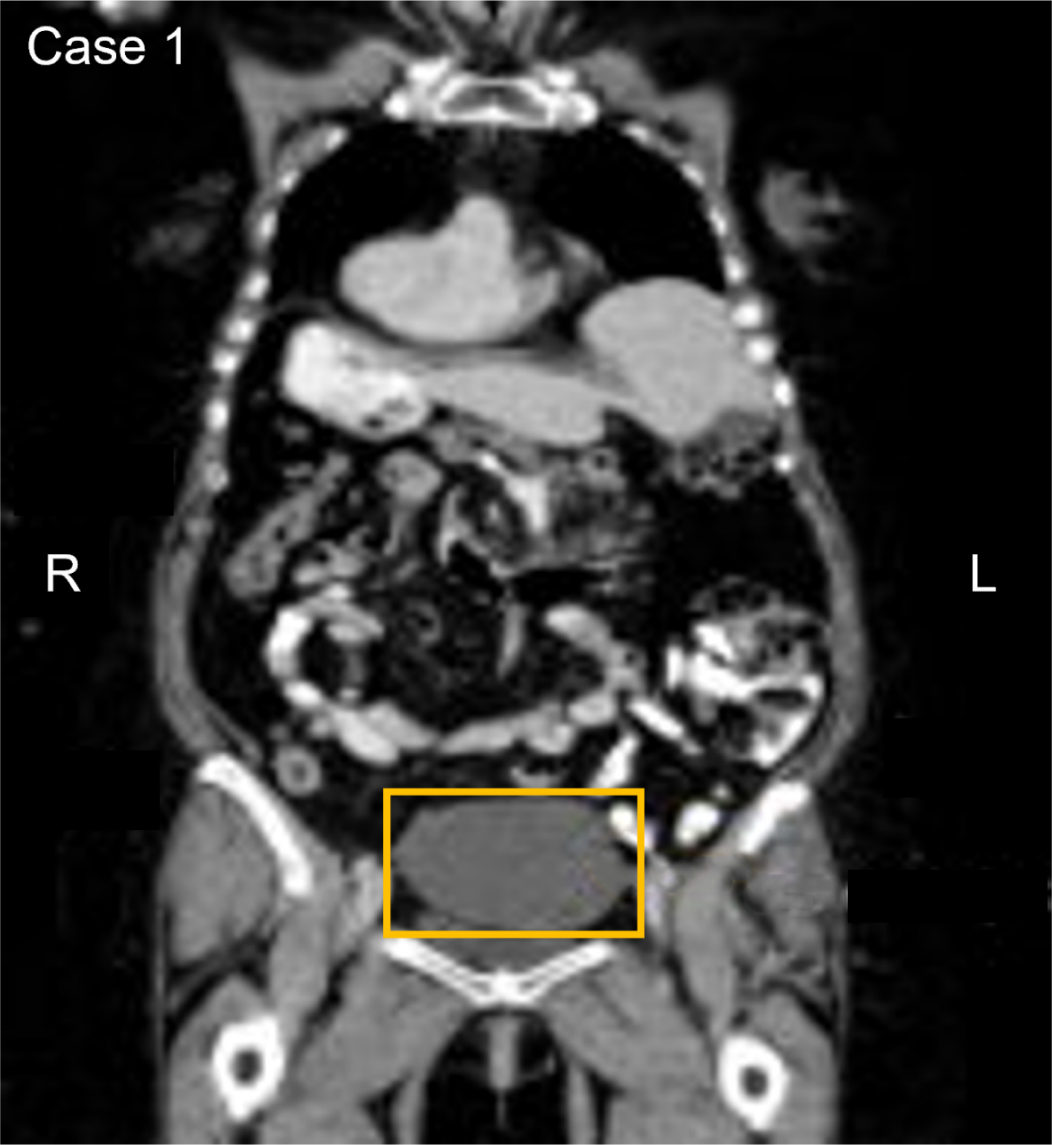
Participant one CT image. The area in the yellow box shows a large cystic mass in the pelvis measuring 10.3 x 9.8 x 7.8 cm.
